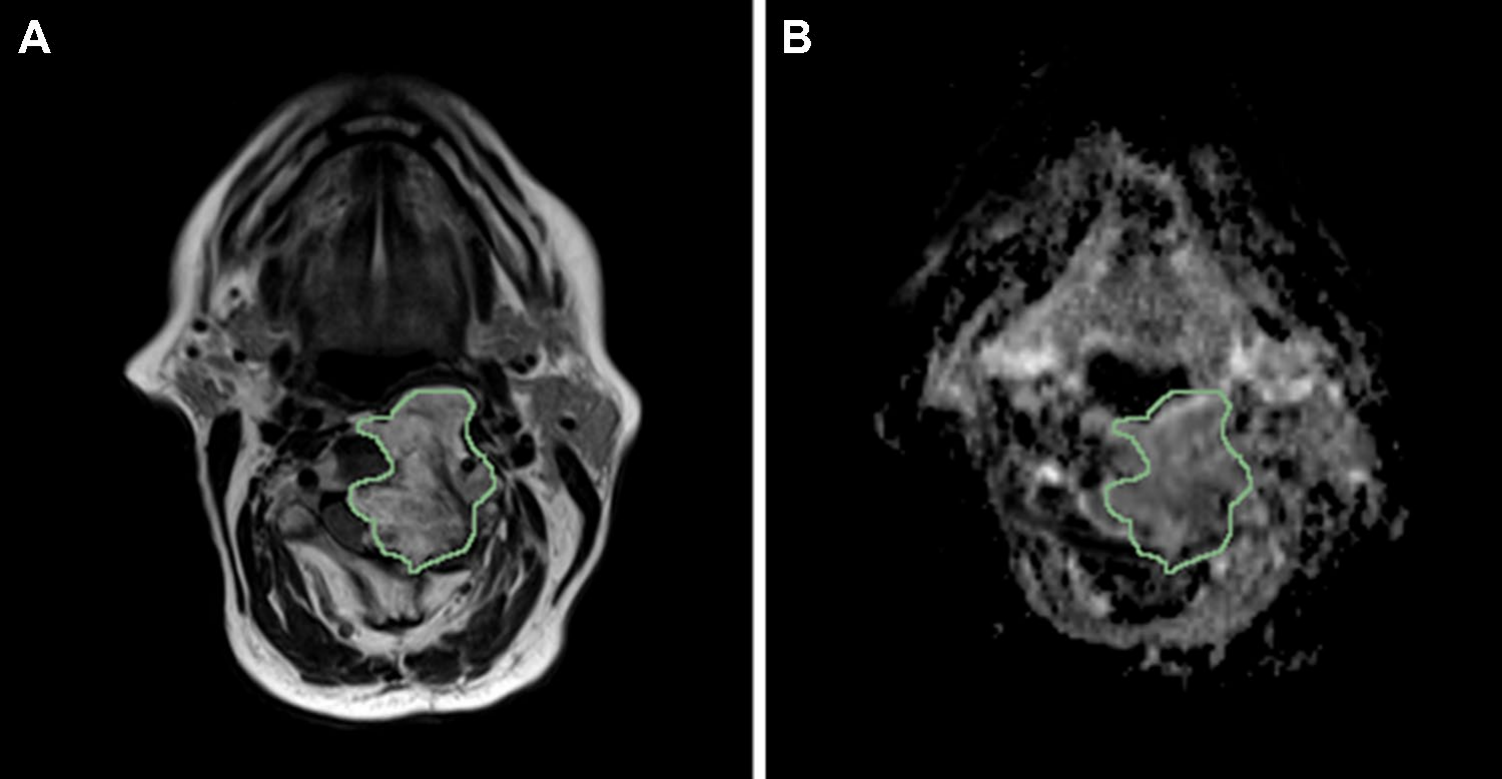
Example of tumor segmented by the radiologist. The tumor is segmented on the T2-weighted image (a) but the same segmentation has been used as a mask to extract the radiomic features from the apparent diffusion coefficient map (b) as well
